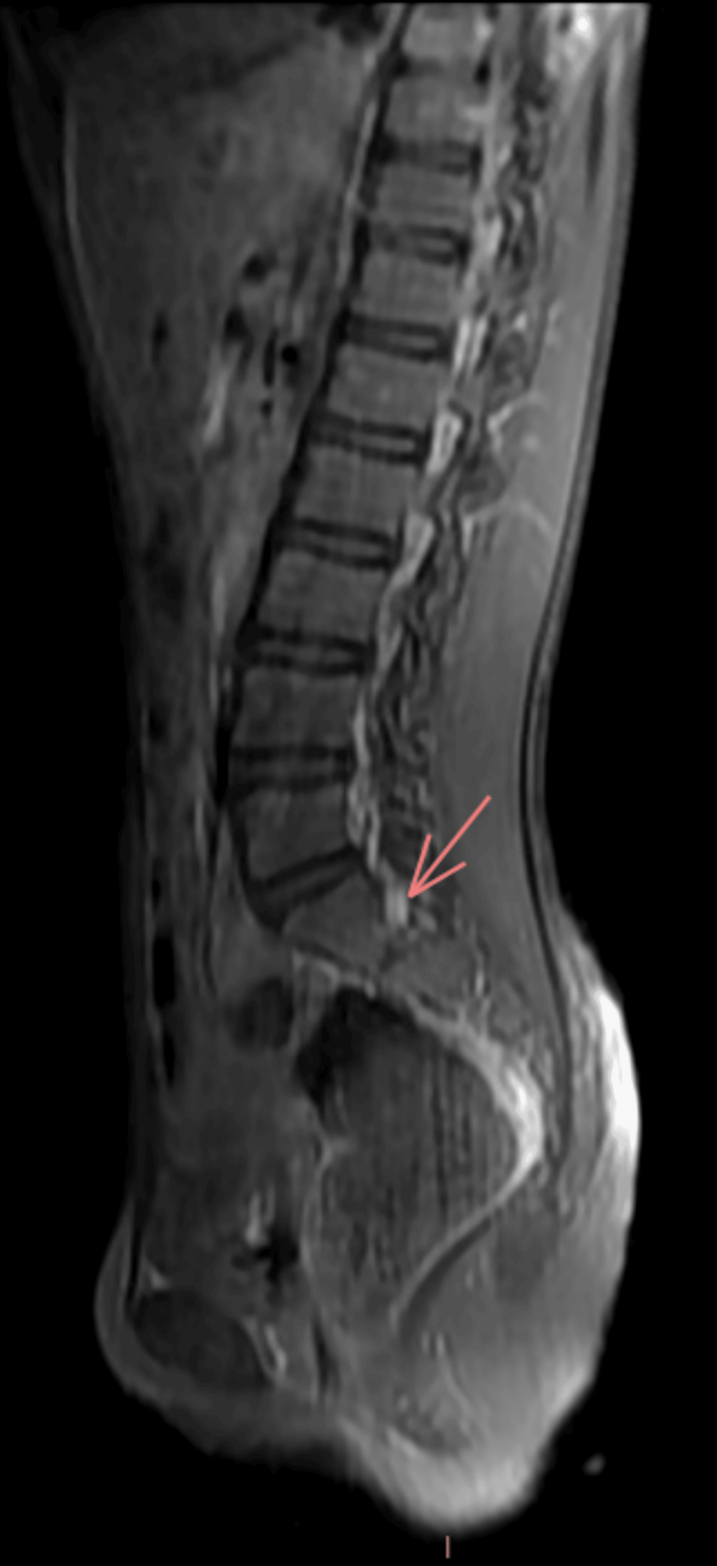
MRI with sagittal section in T1-weighted images with fat suppression and gadolinium contrast Brain and spinal MRI of our patient demonstrates homogeneous and diffuse thickening of the cauda equina, including the lumbar and sacral nerve roots, which appear swollen and show intense enhancement after gadolinium injection. The overall appearance is compatible with Guillain-Barré syndrome.
